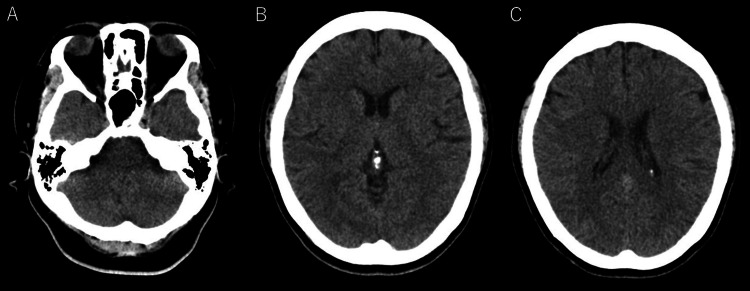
(A-C) Initial head computed tomography showing no evidence of hemorrhage and early ischemic signs.
